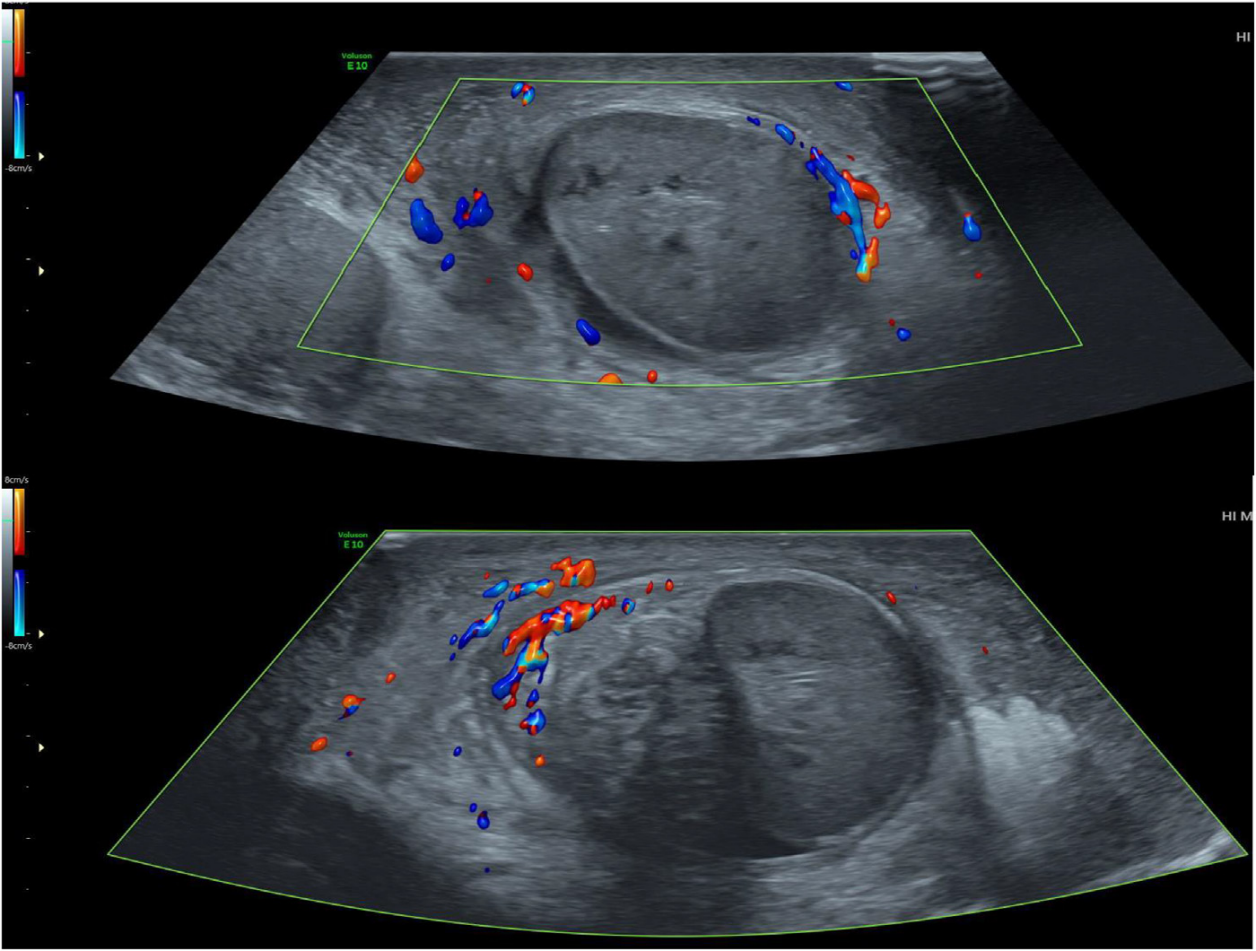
Color Doppler study of the left testicle, objectifying the absence of vascularization of the testicle.
